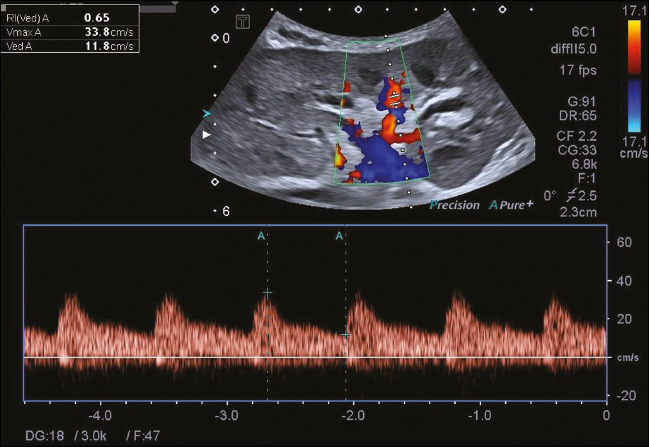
Spectral color Doppler for evaluation of the RI of the interlobar artery.
